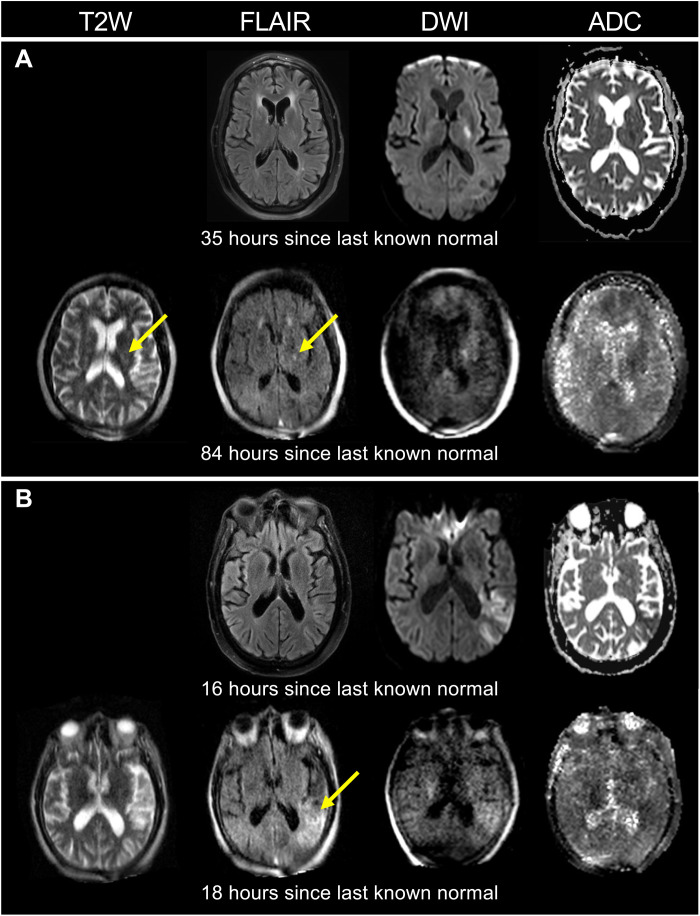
Monitoring of ischemic stroke development with pMRI. (A) A 66-year-old male with a left internal capsule stroke (4-mm infarct). A pMRI exam 49 hours after the initial SOC MRI (3 T) exam demonstrated the interval development of hyperintensity on the T2W and FLAIR sequences (yellow arrows). (B) A 90-year-old male with a left middle cerebral artery stroke. A pMRI exam 2 hours after the initial SOC MRI (3 T) exam revealed the interval development of hyperintensity on the FLAIR sequence (yellow arrow). The SOC MRI exams did not include the T2W sequence.
